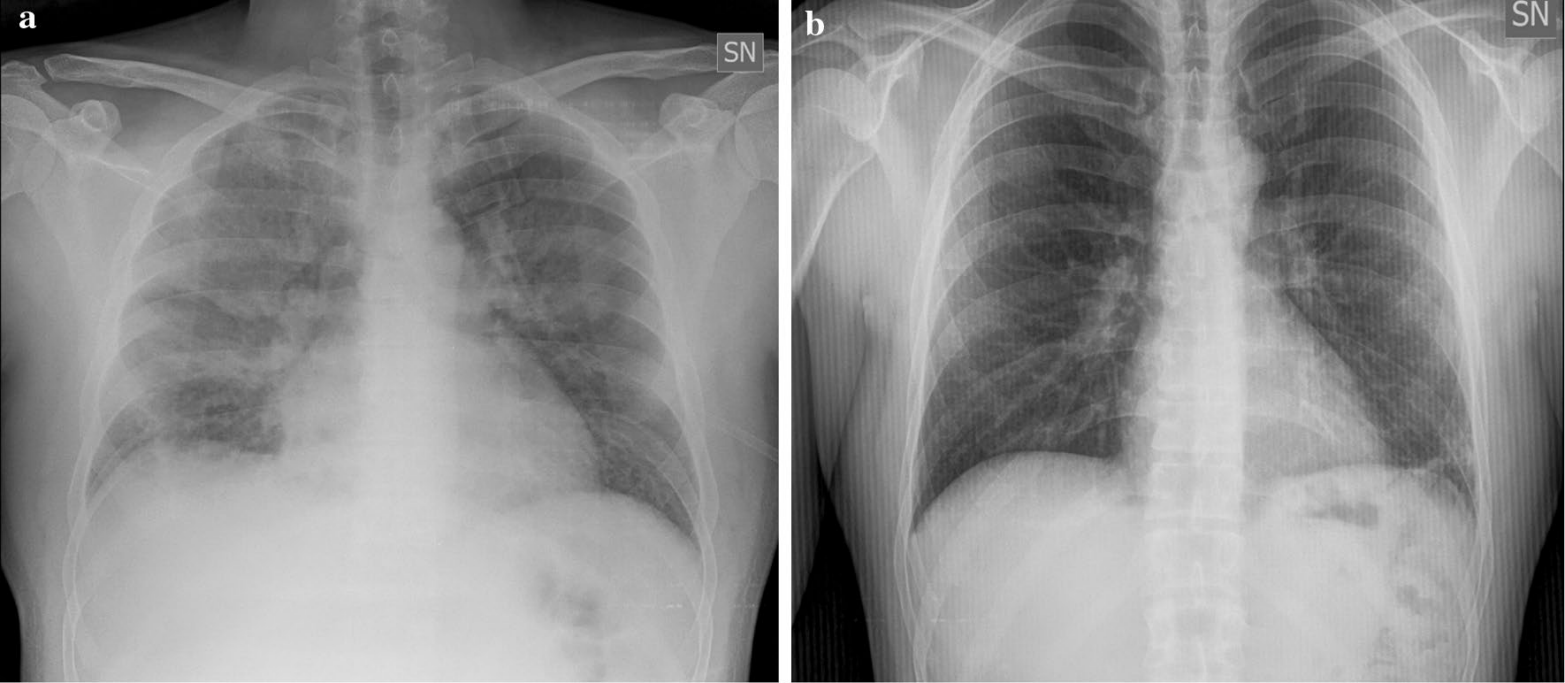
Chest X-ray before (a) and after (b) malaria *Plasmodium ovale* disease. a Chest X-ray showed interstitial bilateral pneumonia; b unremarkable results during the follow-up visit after 2 months
